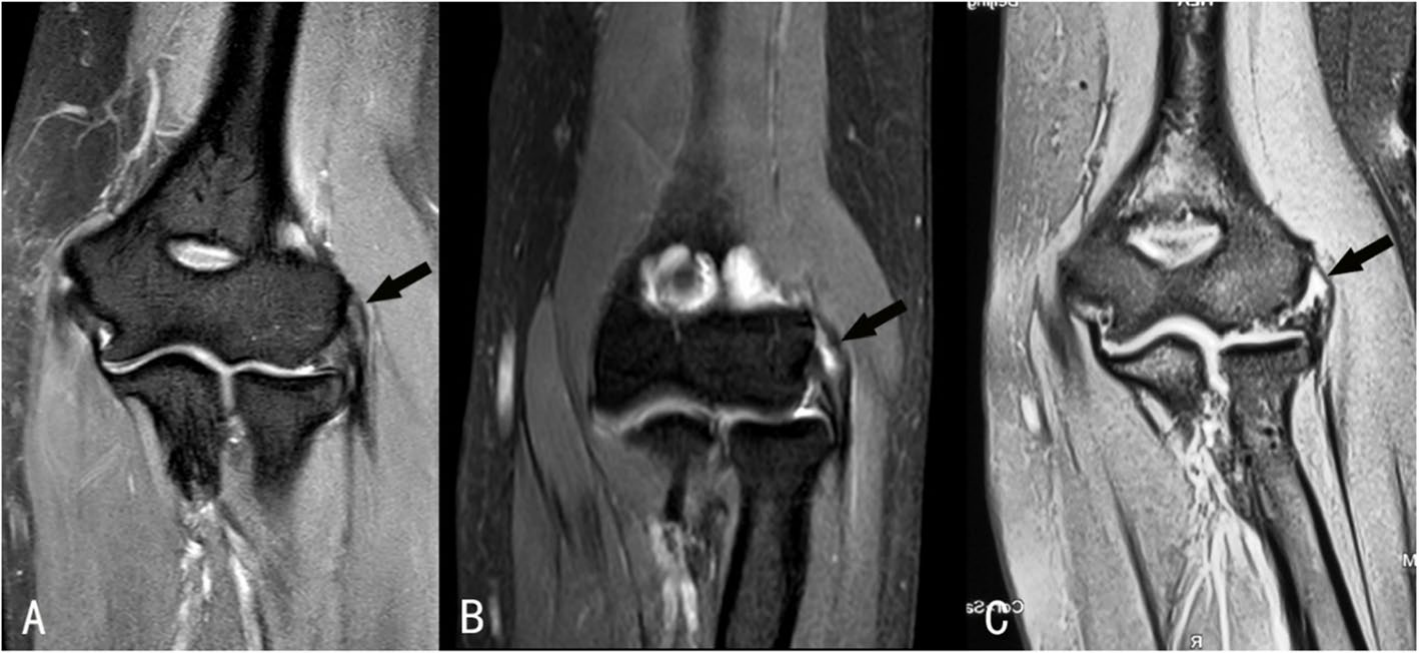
Examples of MRI findings on the origin of the ERCB, the grades are same within the three classifications. A 44-year-old male, MRI showed grade 1 injury on the origin of the ERCB (focal or mild increased signal intensity). B 48-year-old female, MRI detected grade 2 injury on the origin of the ERCB (Moderate increased signal intensity, comprise less than 50% of the tendon thickness). C 52-year-old female, MRI detected grade 3 injury on the origin of the ERCB (Severe increased signal intensity, comprise more than 80% of the tendon thickness) (black arrow)
